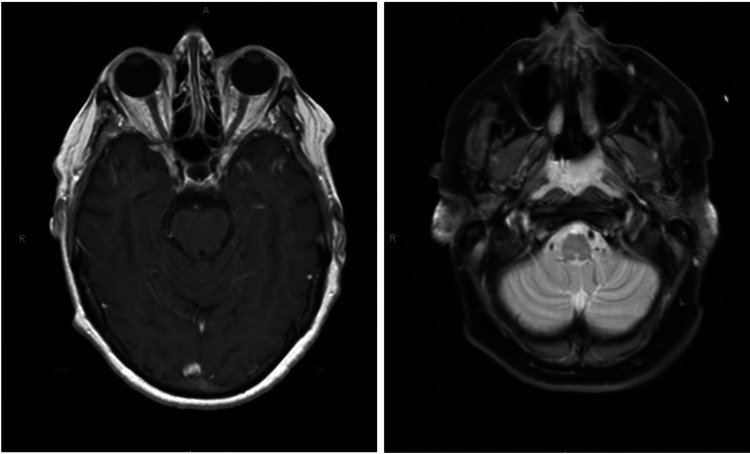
Brain MRI demonstrating chronic ischemic changes. MRI: magnetic resonance imaging.
